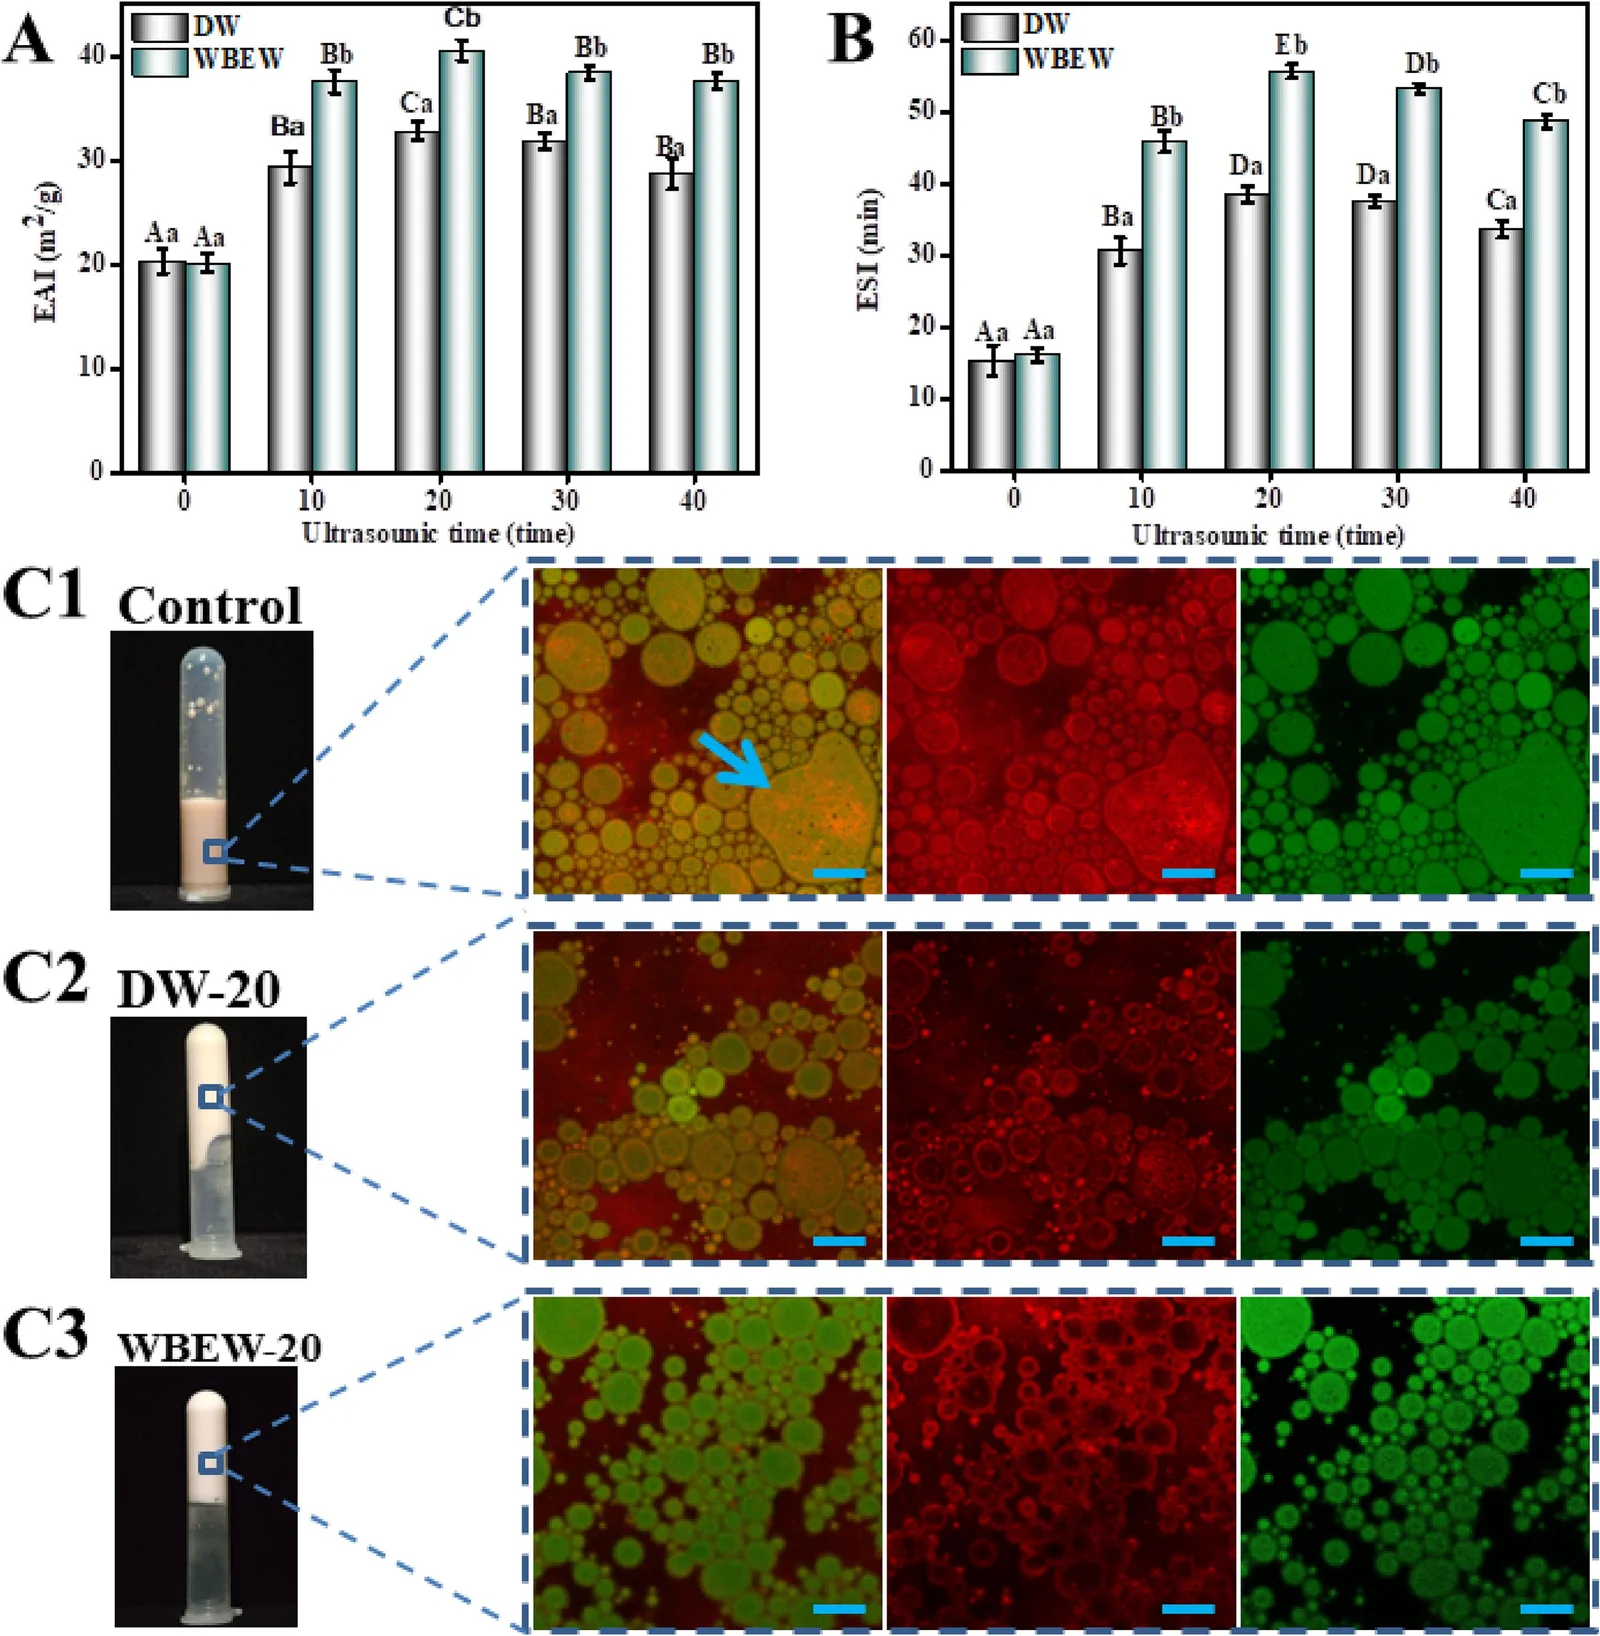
The emulsifying activity index (A) and emulsifying stability index (B) and CLSM images (C) of HIPEs stabilized by native AKP, AKP–GA complexes treated with ultrasound in DW or WBEW AKP–GA complexes. For CLSM images: green = protein (Nile Blue), red = fat (Nile Red). Scale bars = 530  μm.
